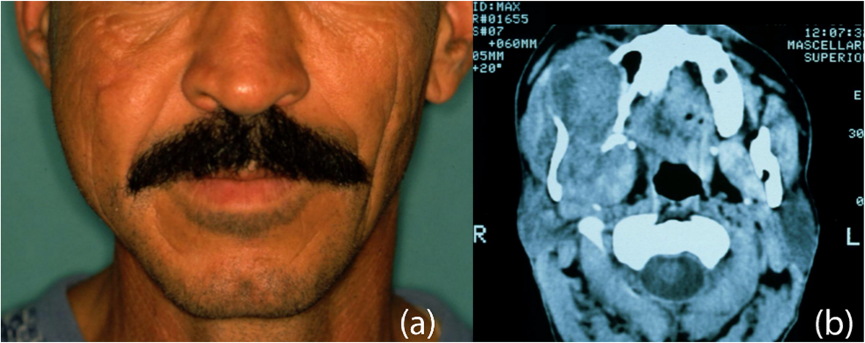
Preoperative patient’s study. (a) Patient’s facial appearance. (b) Axial computed tomography scan showing the extent of the tumor.
